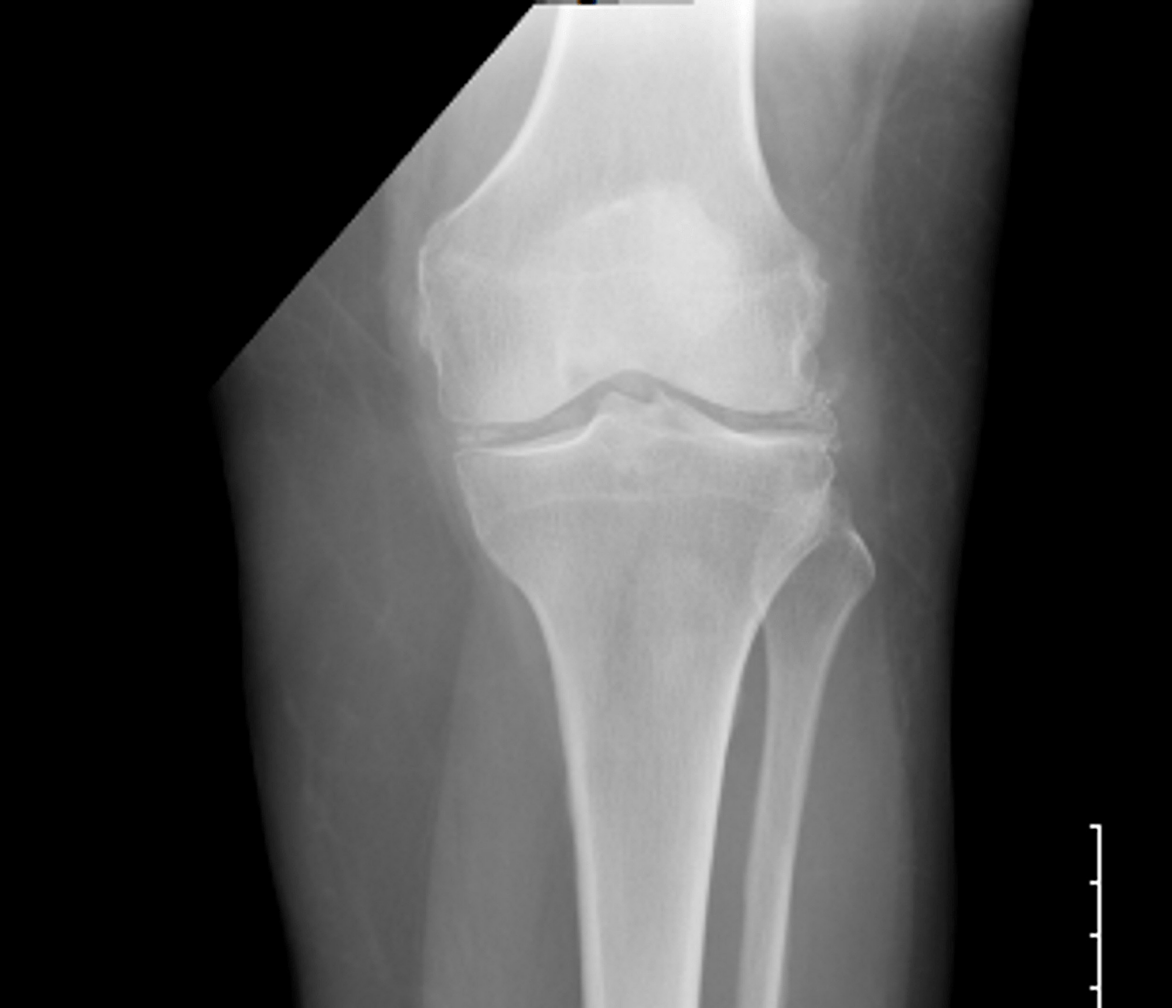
X-ray of the left knee joint showing chondrocalcinosis on the medial and lateral aspects
